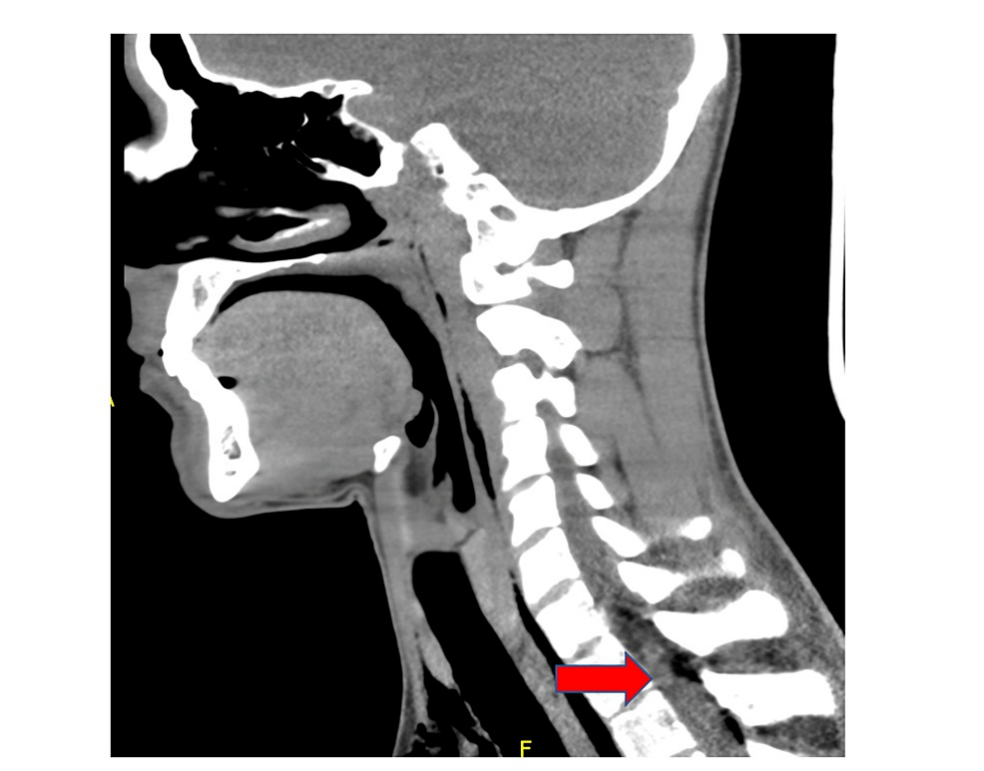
CT soft tissue neck without contrast showing soft tissue emphysema tracking from the mediastinum into the neck and air in the spinal canal (PR).
